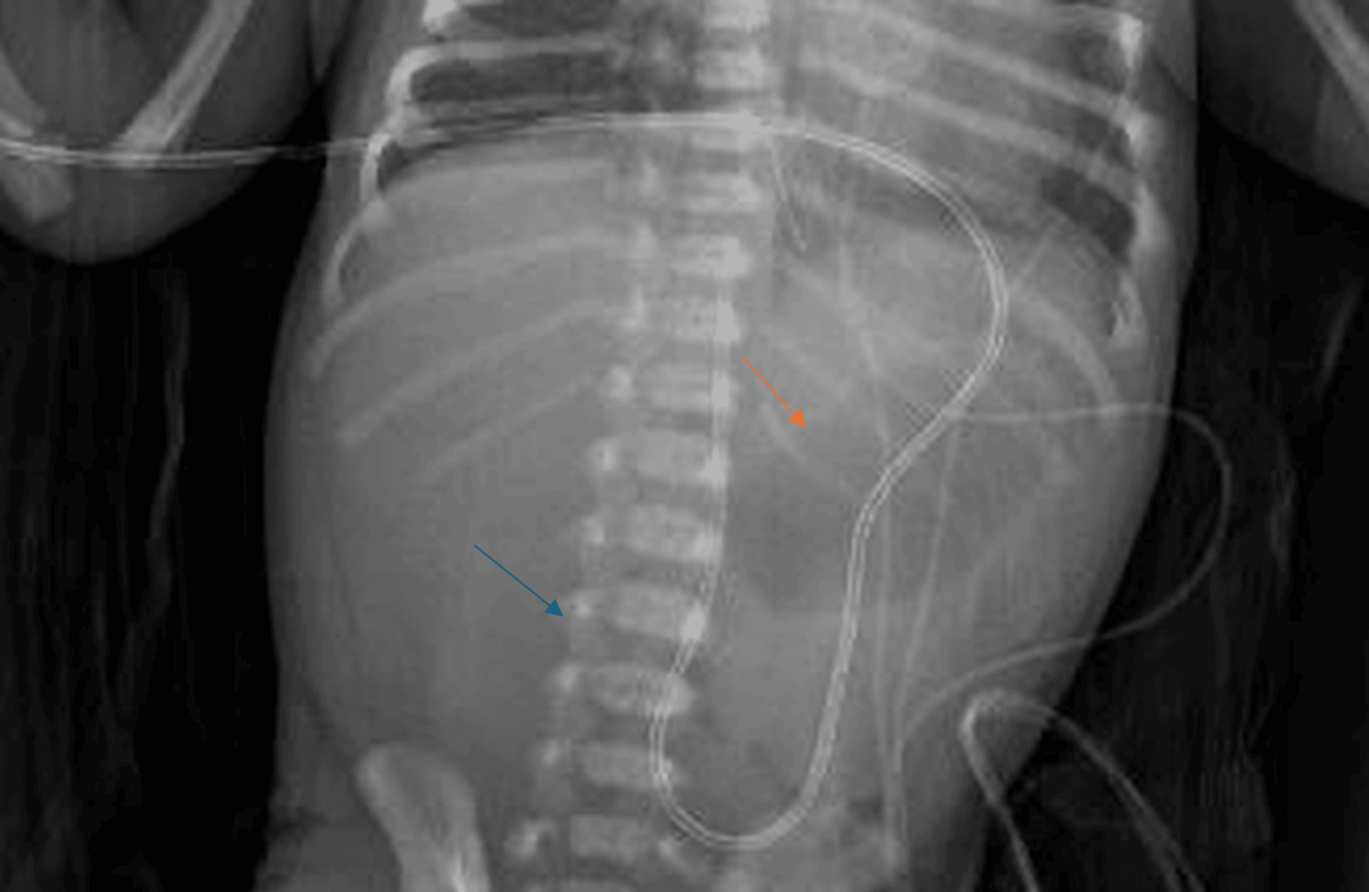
Abdominal X-ray showing abnormal bowel gas pattern The orange arrow points to the prominent gastric gas bubble (dilated stomach), and the blue arrow points to an area of the abdomen with paucity of distal bowel gas
